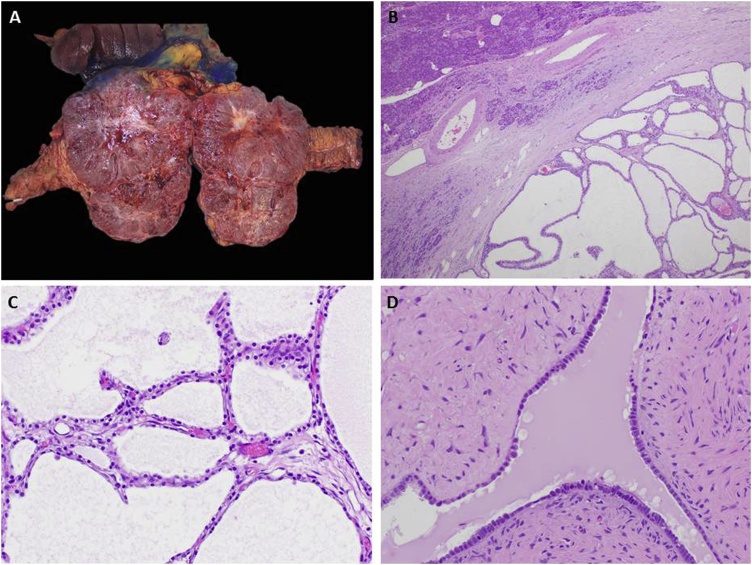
Serous cystadenoma. (A) Well-defined, polycystic mass involving the head of the pancreas. This gross appearance is seen as sponge-like or “honeycomb” on imaging. Note the central stellate scar and the delicate septa which can also be identified on imaging. (B) Low magnification shows a well circumscribed mass, adjacent to uninvolved pancreas (top left), with multiple, back to back, small thin-walled cysts filled with clear serous fluid. Interestingly, the cysts do not communicate with the pancreatic duct as seen in mucinous cystic neoplasms. (C) Rich capillary network contributes to the enhancement on CT imaging. (D) The epithelium lining the cystic spaces is composed of uniform columnar cells with pale cytoplasm rich in glycogen, without atypia or mitotic activity. This bland cytomorphology of a benign neoplasm can be deceiving in cases of serous cystadenocarcinoma, an extremely rare but morphologically indistinguishable tumor from serous cystadenoma.
